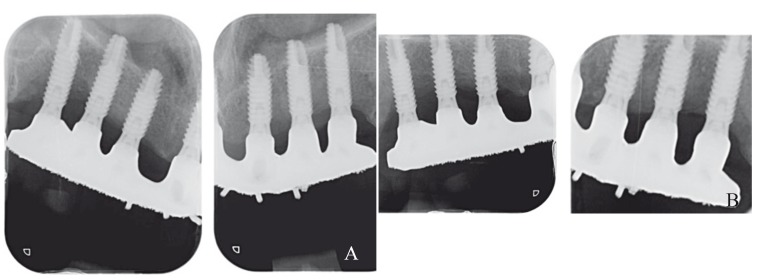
a, b) Periapical radiograph taken at (a) implant loading and (b) the last control visit (6 years post-loading).
